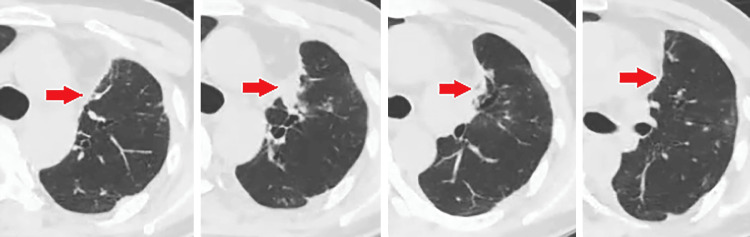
Postoperative contrast-enhanced CT findings Contrast-enhanced CT performed one year after lobectomy showed inflammatory changes at the pneumatocele site (arrow), but no pneumatocele was detected.
